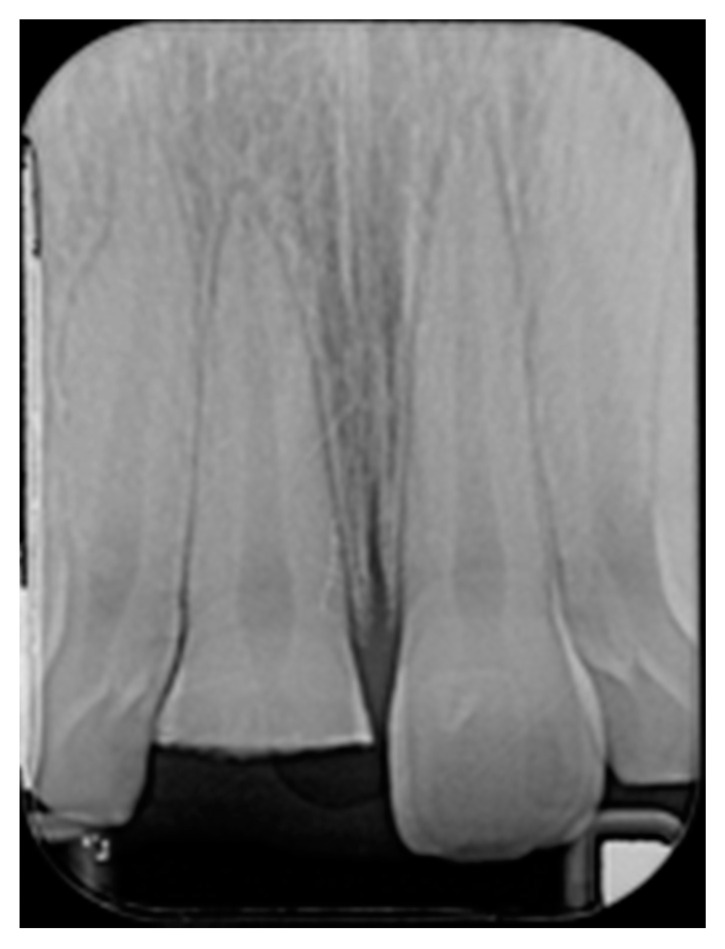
Initial x-ray. Reprinted from Restauri diretti nei settori anteriori, G. Paolone, S. Scolavino, © 2021, with permission from Quintessence Publishing Italy.
